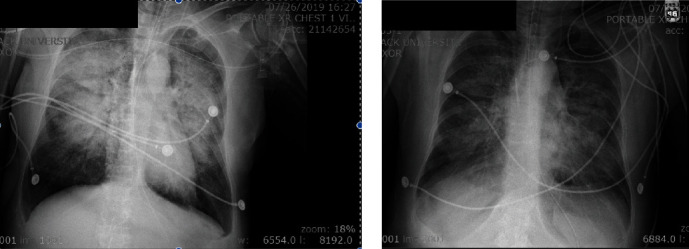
Chest X-ray prior to and following tocilizumab. Improvement of bilateral ground glass opacities following interleukin-6 receptor inhibitor.
